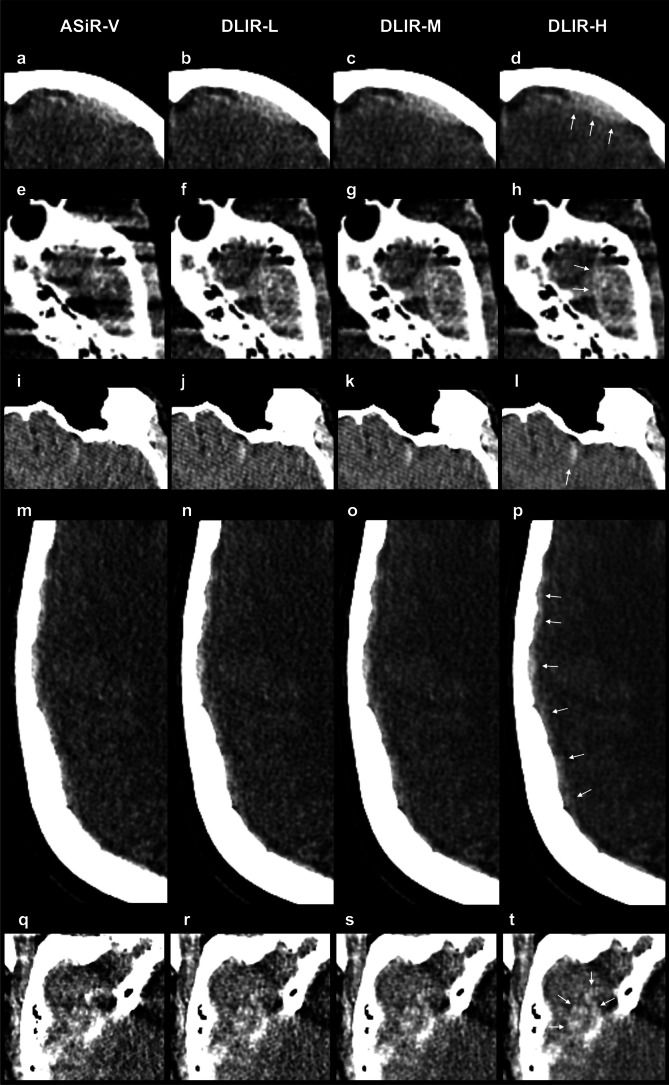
Axial noncontrast trauma head CT images with intracranial hemorrhages, reconstructed with ASiR-V (a, e, i, m, q), DLIR-L (b, f, j, n, r), DLIR-M (c, g, k, o, s), and DLIR-H (d, h, l, p, t). The first row of images (a–d) is of a 26-year-old female patient who was assaulted. A left frontal epidural hematoma was diagnosed (white arrows in image d). The second row of images (e–h) is of a 22-year-old male that was involved in a motor vehicle accident. At the level of the pons/interpetrous region, an epidural hematoma in the middle cranial fossa on the left side was diagnosed (white arrows in image h). The third row of images (i–l) is of a 33-year-old male, a pedestrian who was hit by a car. A left frontal subarachnoid hemorrhage was diagnosed (white arrow in image l). The fourth row of images (m–p) is of a 62-year-old female who fell at home. An acute subdural hematoma along the right cerebral convexity was diagnosed (white arrows in image p). The fifth row of images (q–t) is of a 69-year-old male who fell. Cerebral hemorrhagic contusions were diagnosed adjacent to the floor of the right middle cranial fossa (white arrows in image t), and all three readers graded the ASiR-V images as non-diagnostic (score 1 or 2) and the DLIR-H images as diagnostic (score > 2). Overall, regarding intracranial hemorrhage conspicuity, the readers graded fewer CT series as non-diagnostic (score 1 or 2) for DLIR-M and DLIR-H compared to ASiR-V and DLIR-L
